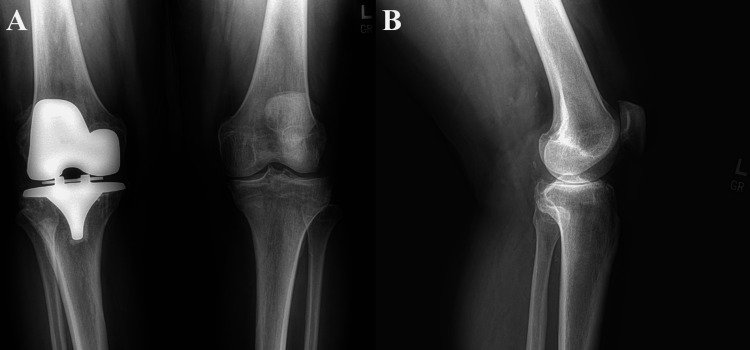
(A) Preoperative anteroposterior radiograph of the knees demonstrating right knee total arthroplasty and left knee osteoarthritis. (B) Preoperative lateral radiograph of the left knee demonstrating osteoarthritis. (A) Preoperative anteroposterior X-ray of both knees demonstrates a well-positioned total knee arthroplasty in the right knee with stable osseous integration and no signs of loosening. The left varus knee demonstrates severe medial compartment osteoarthritis with joint space narrowing, subchondral sclerosis, and osteophyte formation, with no acute fractures identified. (B) Preoperative lateral X-ray of the left knee demonstrates osteoarthritis with joint space narrowing, subchondral sclerosis, and osteophyte formation, with no acute fractures identified.
